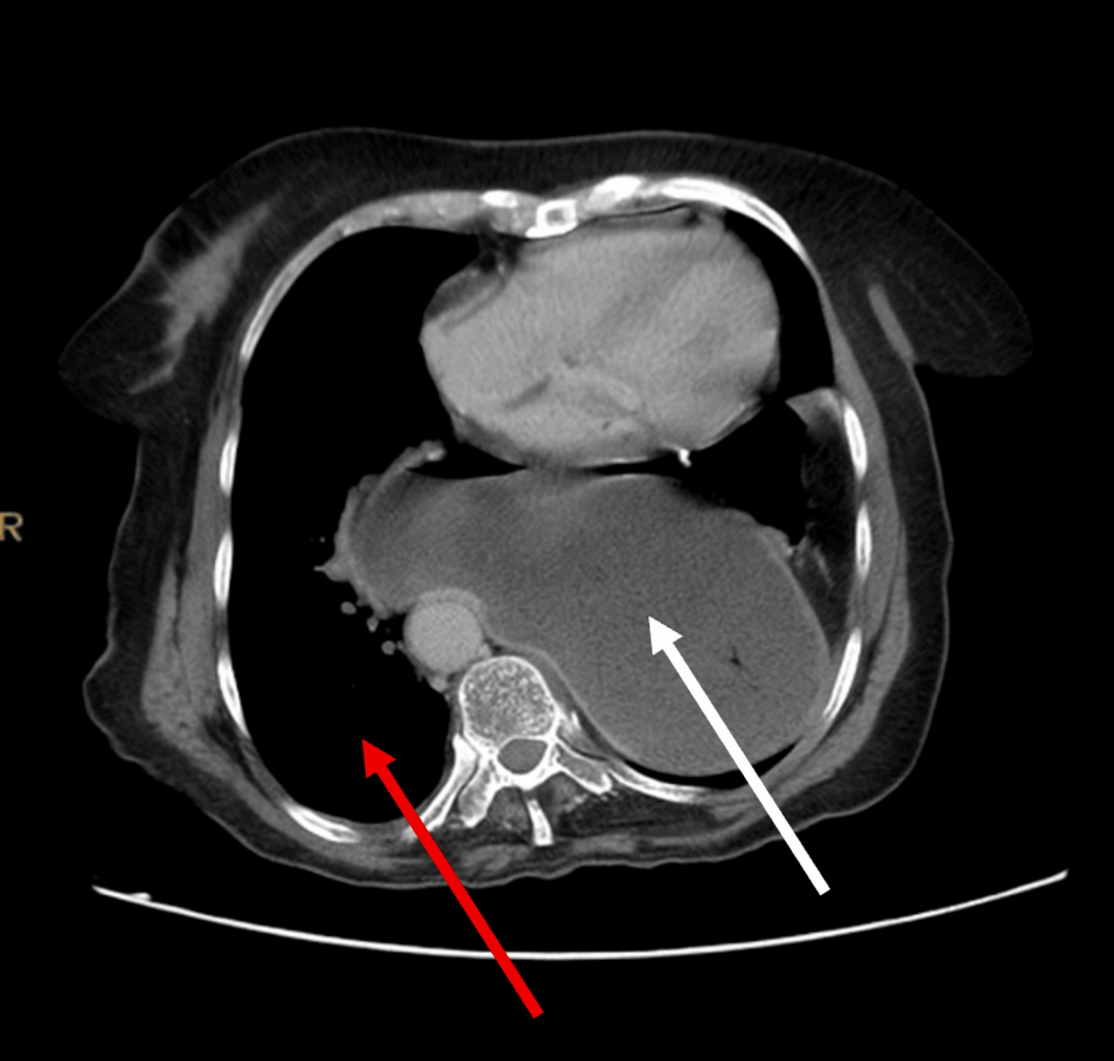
Axial CT Image of the Chest and Abdomen Confirming Giant Hiatal Hernia with Intrathoracic Herniation of Abdominal Viscera. Axial computed tomography (CT) image of the chest and abdomen with contrast demonstrating a giant hiatal hernia (neck diameter 5.2 cm) containing the majority of the stomach (white arrow) and a portion of the left colon (red arrow) herniated into the thoracic cavity posterior to the heart. The herniated viscera occupy a substantial portion of the left hemithorax, causing direct mechanical compression of the left ventricle and adjacent cardiac structures, reduced left lung volume, and leftward displacement of mediastinal contents.
